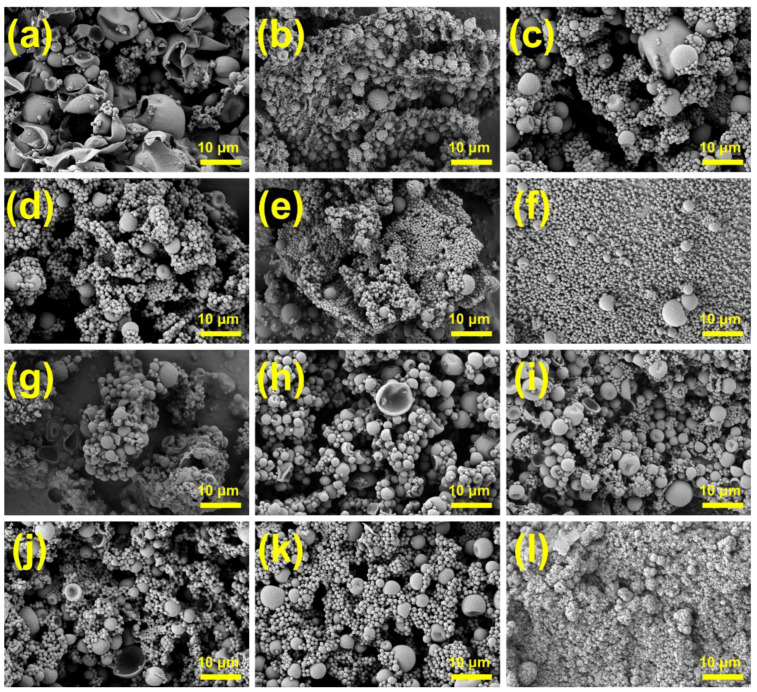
SEM images of MPCMs prepared with different ultrasonic dispersion parameters: (a) 150 W, (b) 225 W, (c) 300 W, (d) 375 W, (e) 450 W, (f) 525 W, (g) 4 min, (h) 6 min, (i) 8 min, (j) 10 min, (k) 450 W, (l) 12 min.
